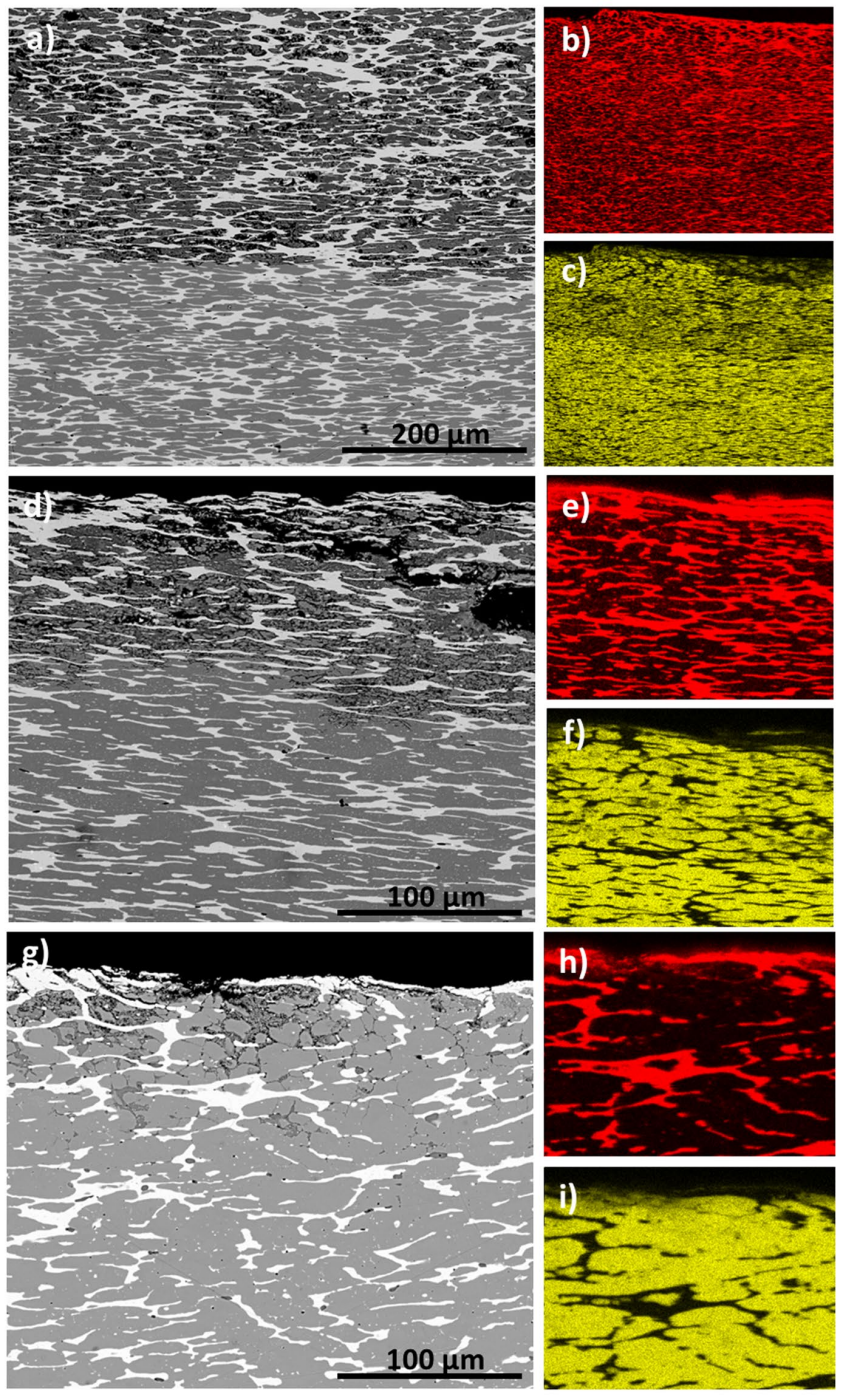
BSE images showing the microstructures of *Antoninianus* coins (a,d,g) C, G and H and their X-ray maps of (b,e,h) Ag and (c,f,i) Cu.
